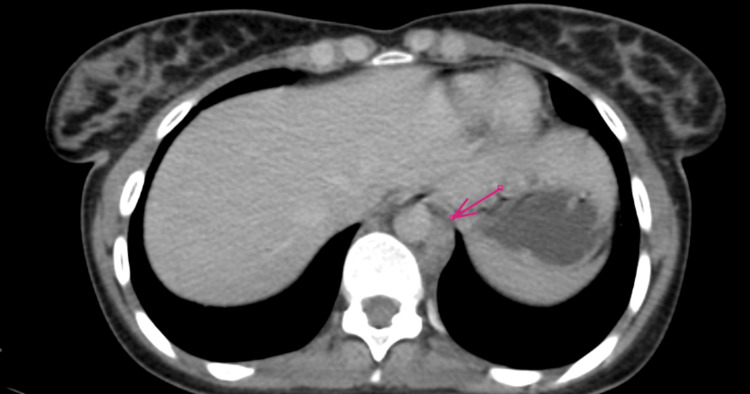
Axial CT scan images (arrow) Showing supradiaphragmatic mass adhering to the descending aorta and abdominal aorta, in close contact with the vertebral bodies of D11 and D12.
